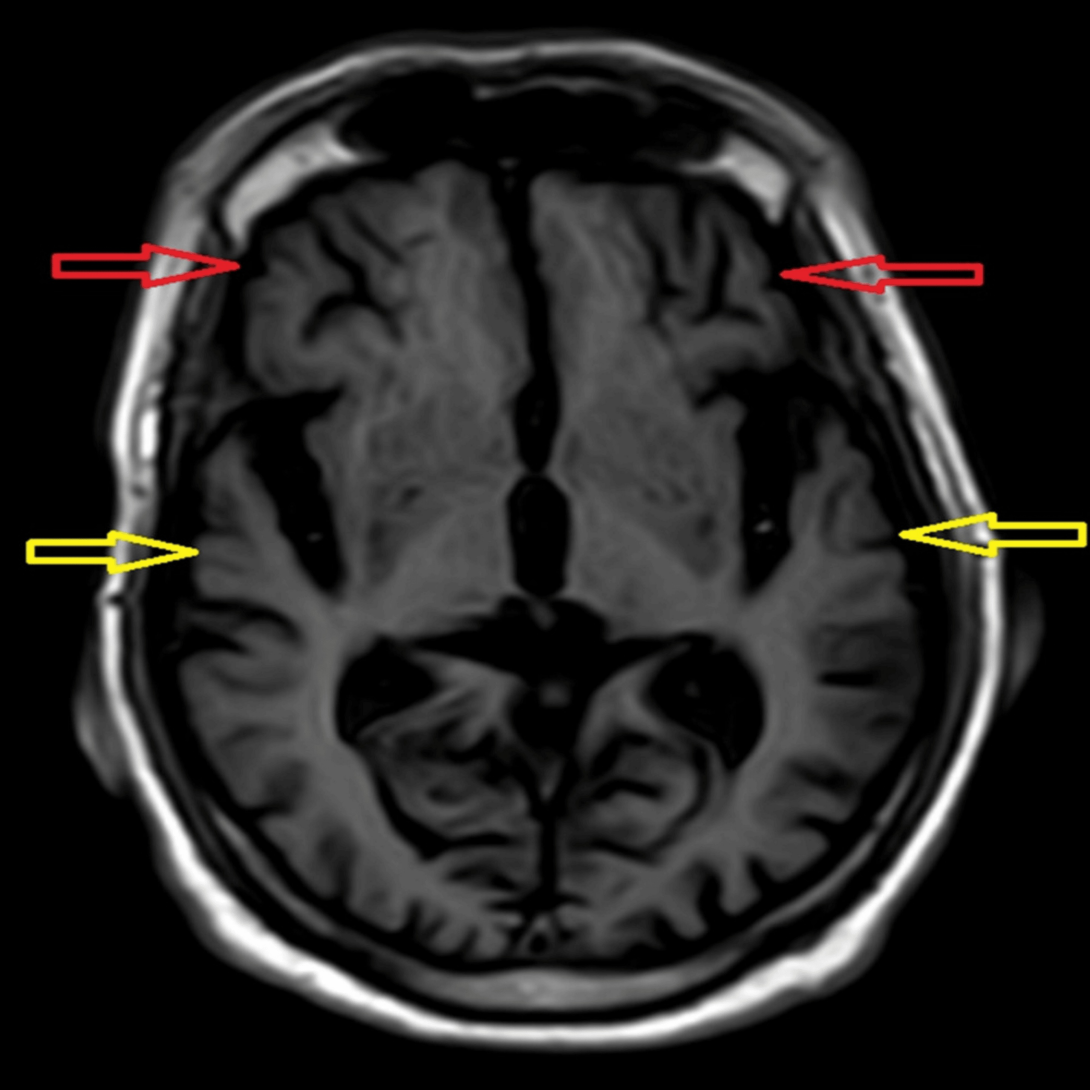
MRI in T1-weighted axial section showing atrophy of the bilateral frontal lobe (red arrows) and temporal lobe (yellow arrows)
